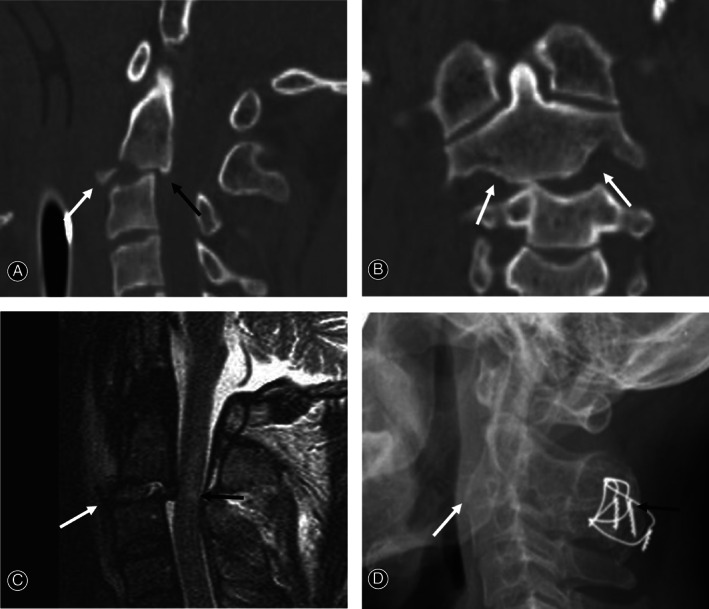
Sagittal computed tomography (CT) scan (A) showing anterior tear drop (TD) fracture (white arrow) and C_2‐3_ posterior slip (dark arrow) without hangman's fracture. Coronal CT scan (B) showing C_2‐3_ bilateral facet dislocation (white arrows). Sagittal magnetic resonance imaging (MRI) (C) showing C_2‐3_ discoligamentous injuries (white arrow) and spinal cord injury with intramedullary hemorrhage (dark arrow). At 24 months follow‐up after skull traction and surgery, lateral X‐ray (D) shows solid fusion of posterior C_2‐3_ fusion (dark arrow) and anterior TD fracture (white arrow).
